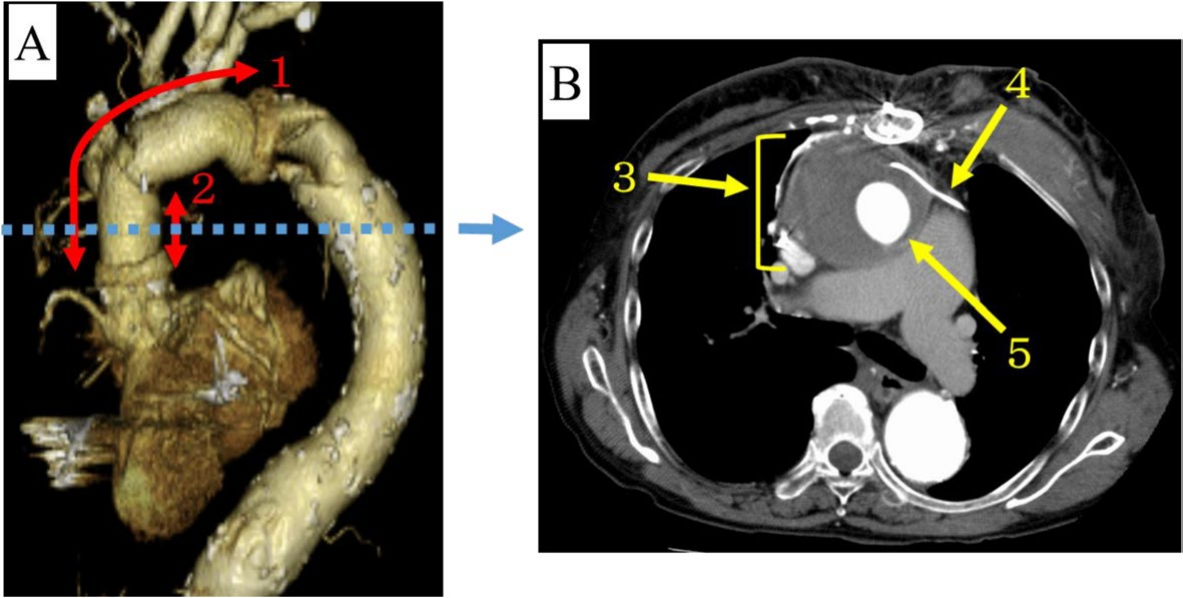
Enhanced computed tomography. A: 3D imaging from left anterior oblique; ①Aortic arch replacement on the first sternotomy. ②Ascending aorta replacement on the second sternotomy. B: Axial view; ③Periprosthetic vascular graft hematoma ④Lead on epicardium. ⑤Prosthetic vascular graft on ascending aorta
